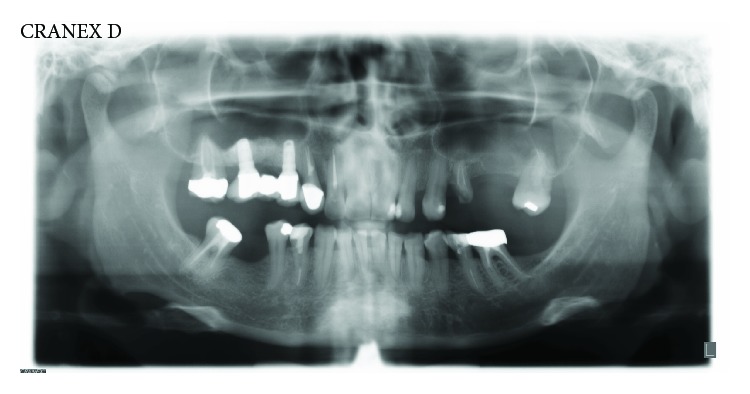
Preoperative panoramic radiograph of the patient.
